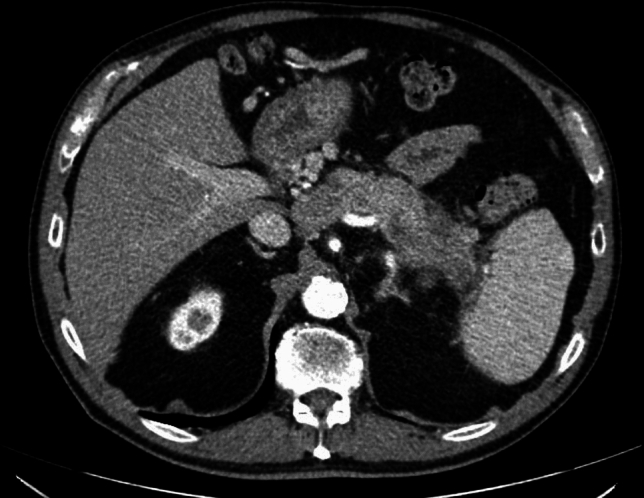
CT demonstrating surrounding inflammatory fat stranding around pancreas consistent with acute pancreatitis
